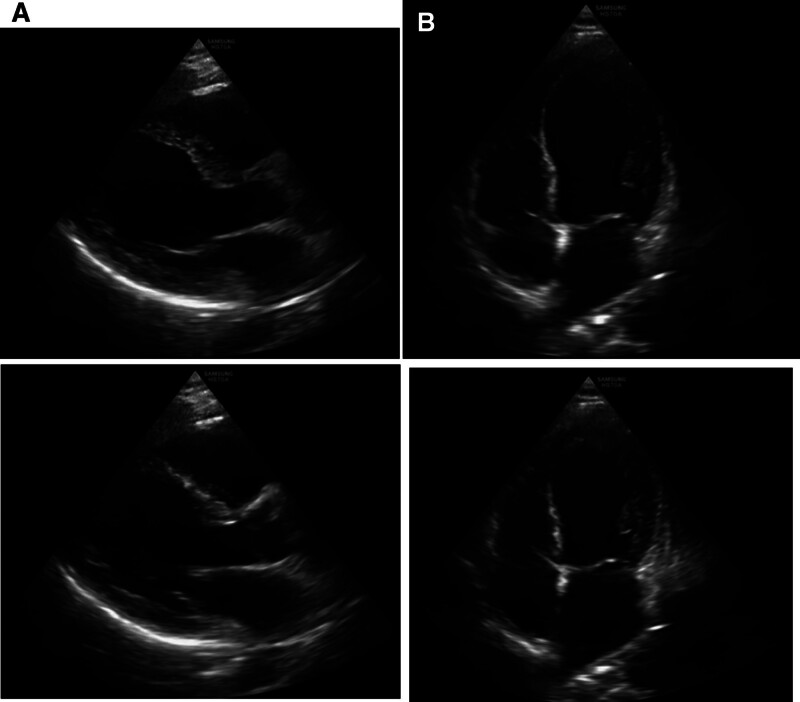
Initial cardiac POCUS of the end-diastolic and end-systolic phases shows regional wall motion abnormality at midportion of left ventricle. (A) Parasternal long-axis view of the end-diastolic (upper) and end-systolic (lower) phases. (B) Apical four-chamber view of the end-diastolic (upper) and end-systolic (lower) phases.
